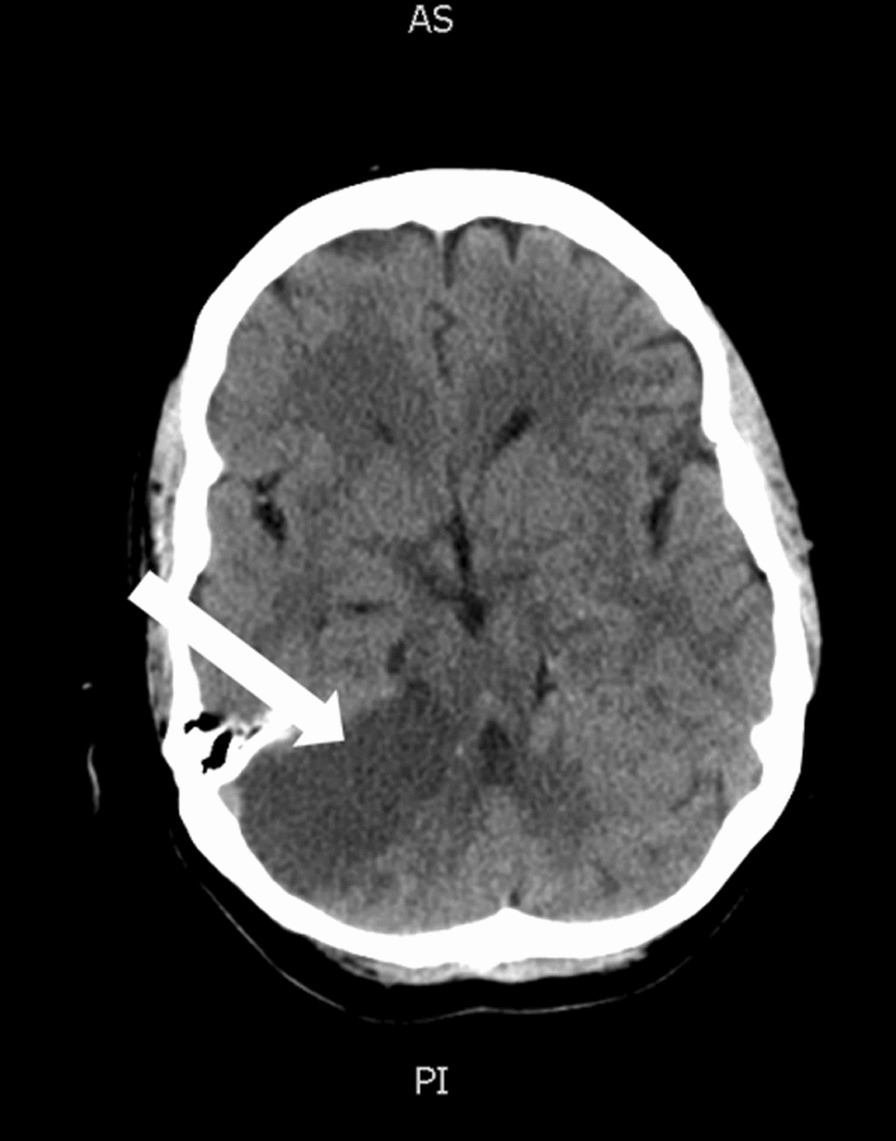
Plain computed tomography brain scan showing ischemia of the lateral right cerebellar hemisphere (arrow)
